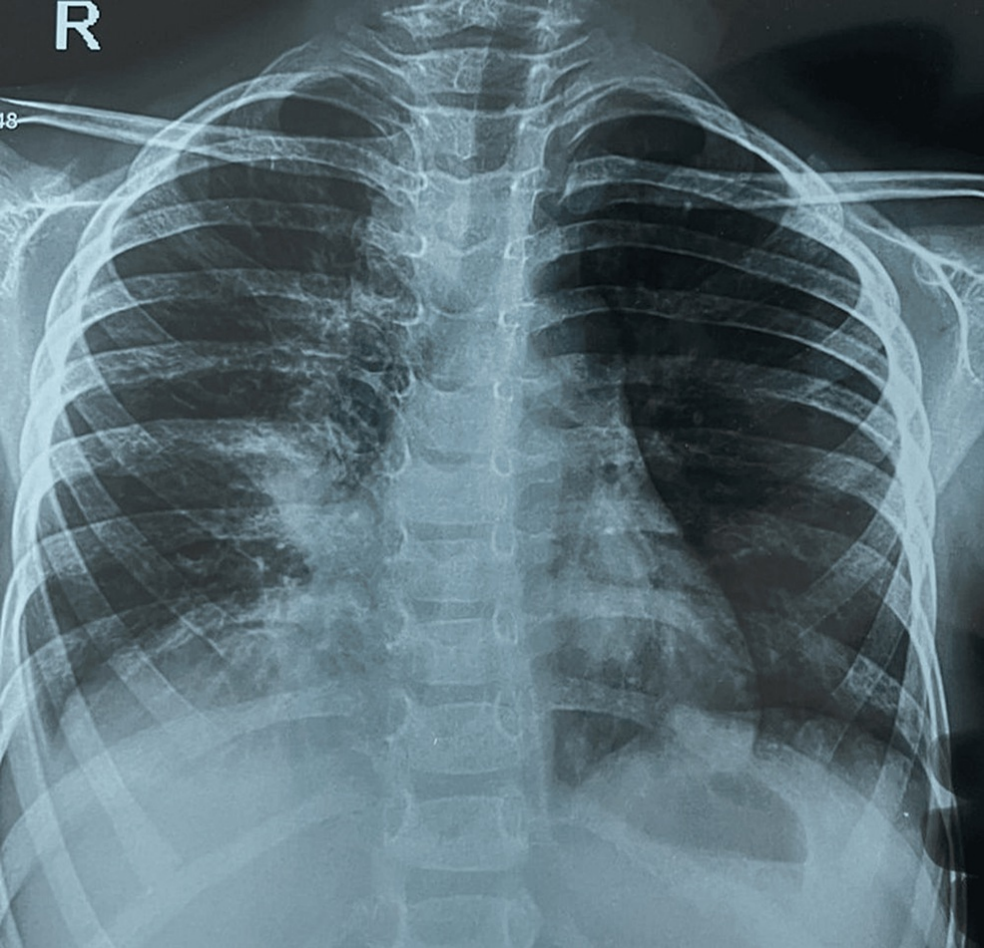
Chest X-ray showing the right middle-lobe consolidation with cardiac, which is silhouetted
